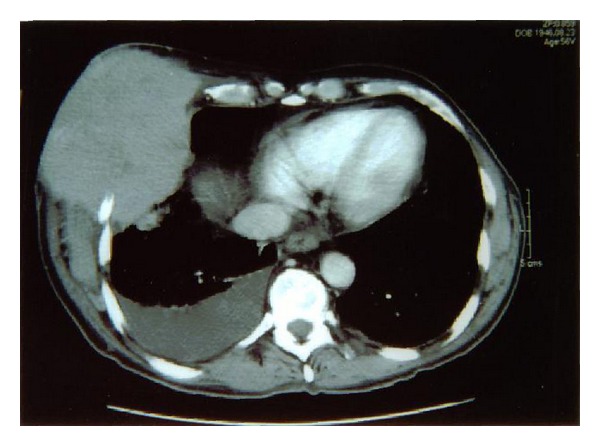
CT chest showing eroding mass lesion.
